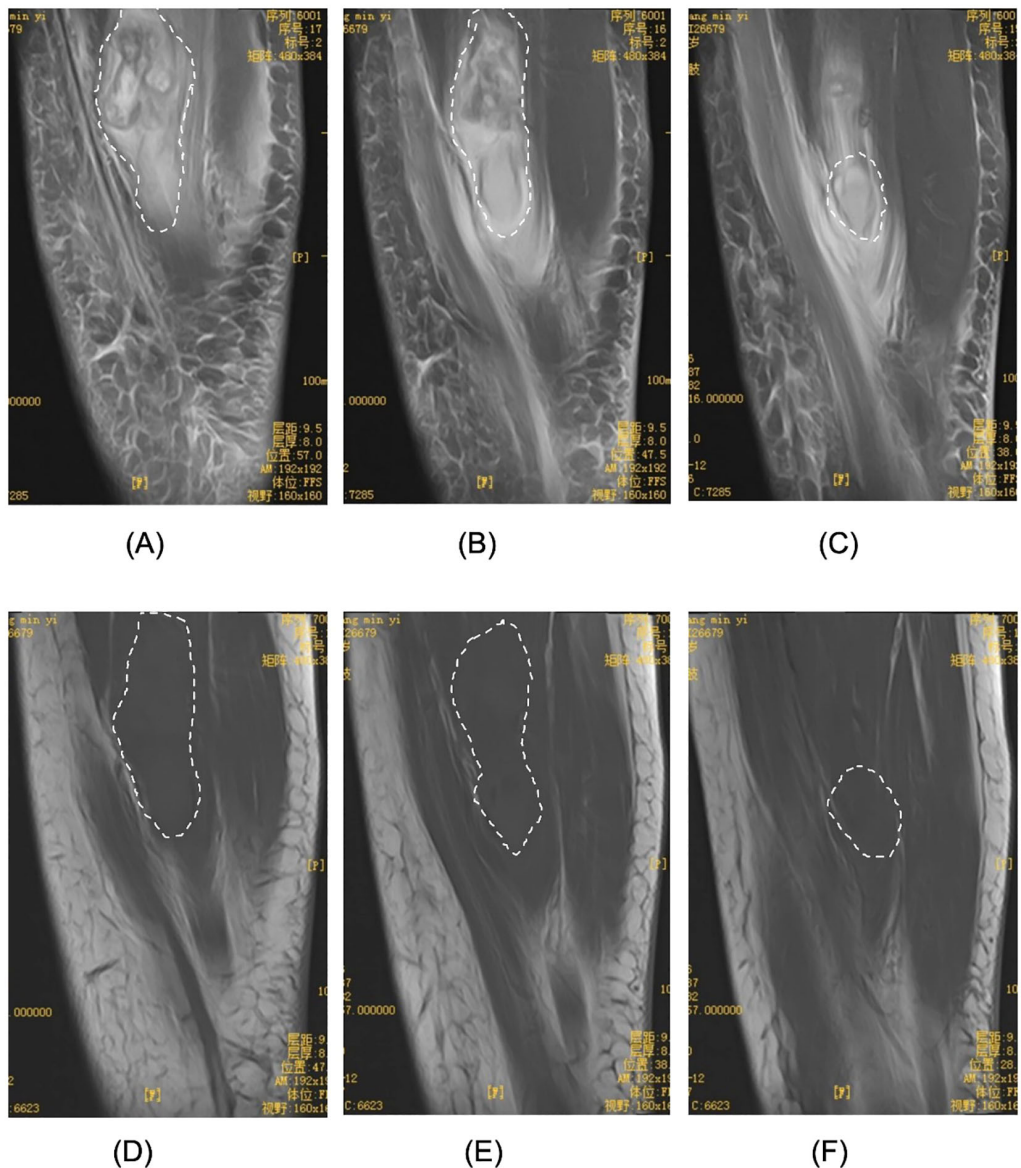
Lower Extremity MRI image of a skeletal muscle tumor in the right thigh. (A-C) on fat-suppressed sequences, they appear hyperintense with thick-walled cystic components and indistinct margin, measuring approximately 2.8 × 3.2 × 9.7 cm. (D-F) T1-weighted images show mildly hypointense signals with irregular contours.
